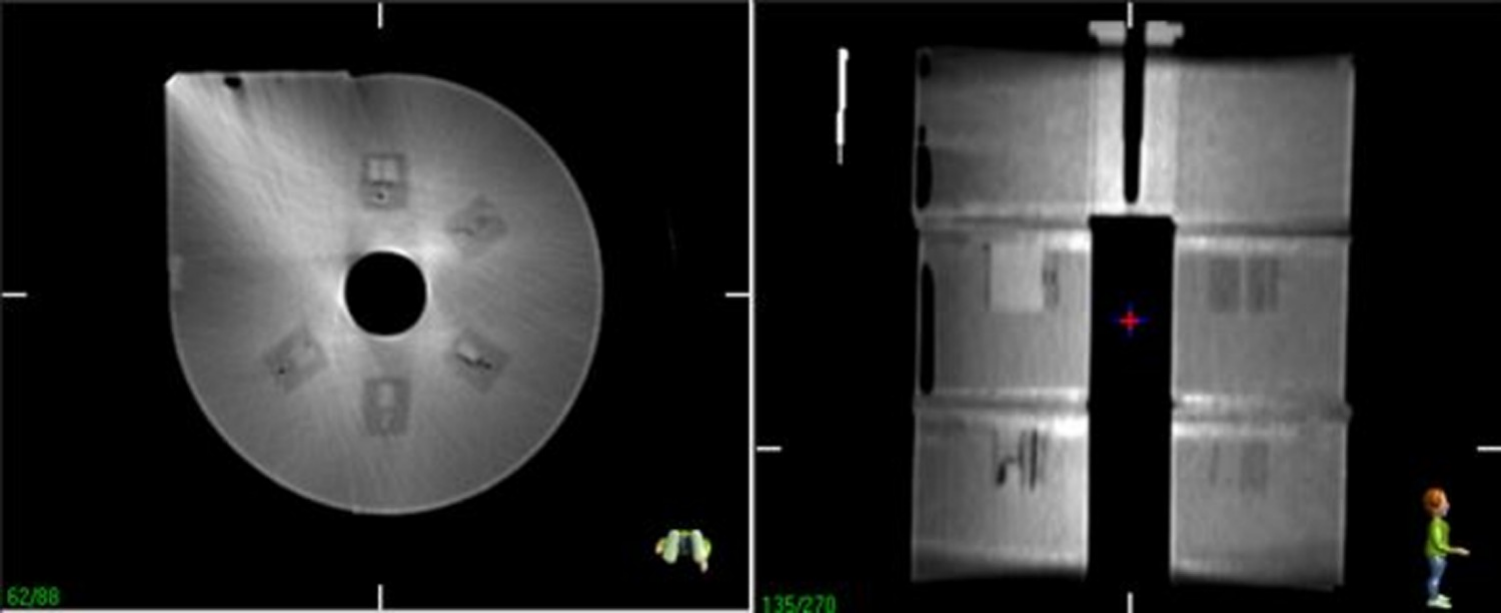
CBCT images of the high-spatial-resolution slab.
